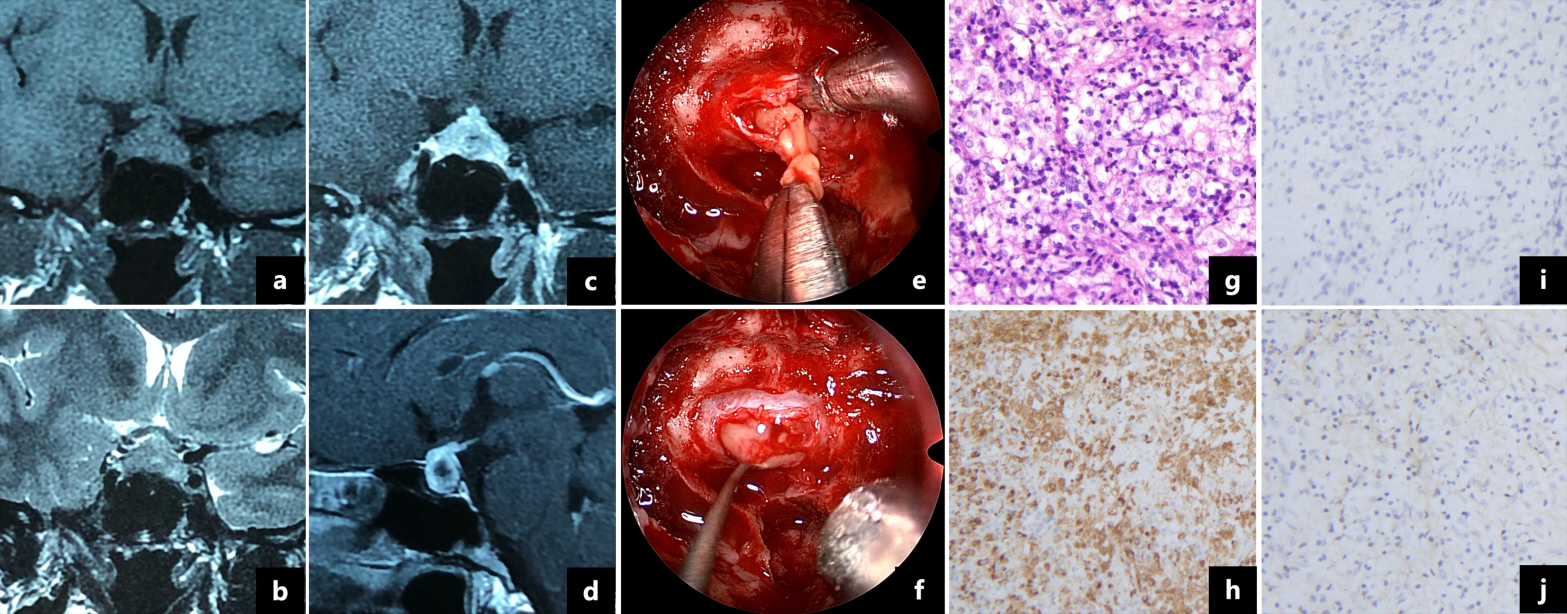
Magnetic resonance imaging showed a 1.7×1.2 cm cystic lesion with a thickened pituitary stalk and mainly peripheral enhancement (A–D). Intraoperative pictures revealed a soft yellow lesion (E) with pus-like fluid (F). Pathologic examination showed that the pituitary gland was infiltrated by foamy histocytes and lymphocytes (G, ×200). The foamy cells were immunopositive for CD68 (H, ×200) and immunonegative for CD1a (I, ×200) and S-100 protein (J, ×200).
